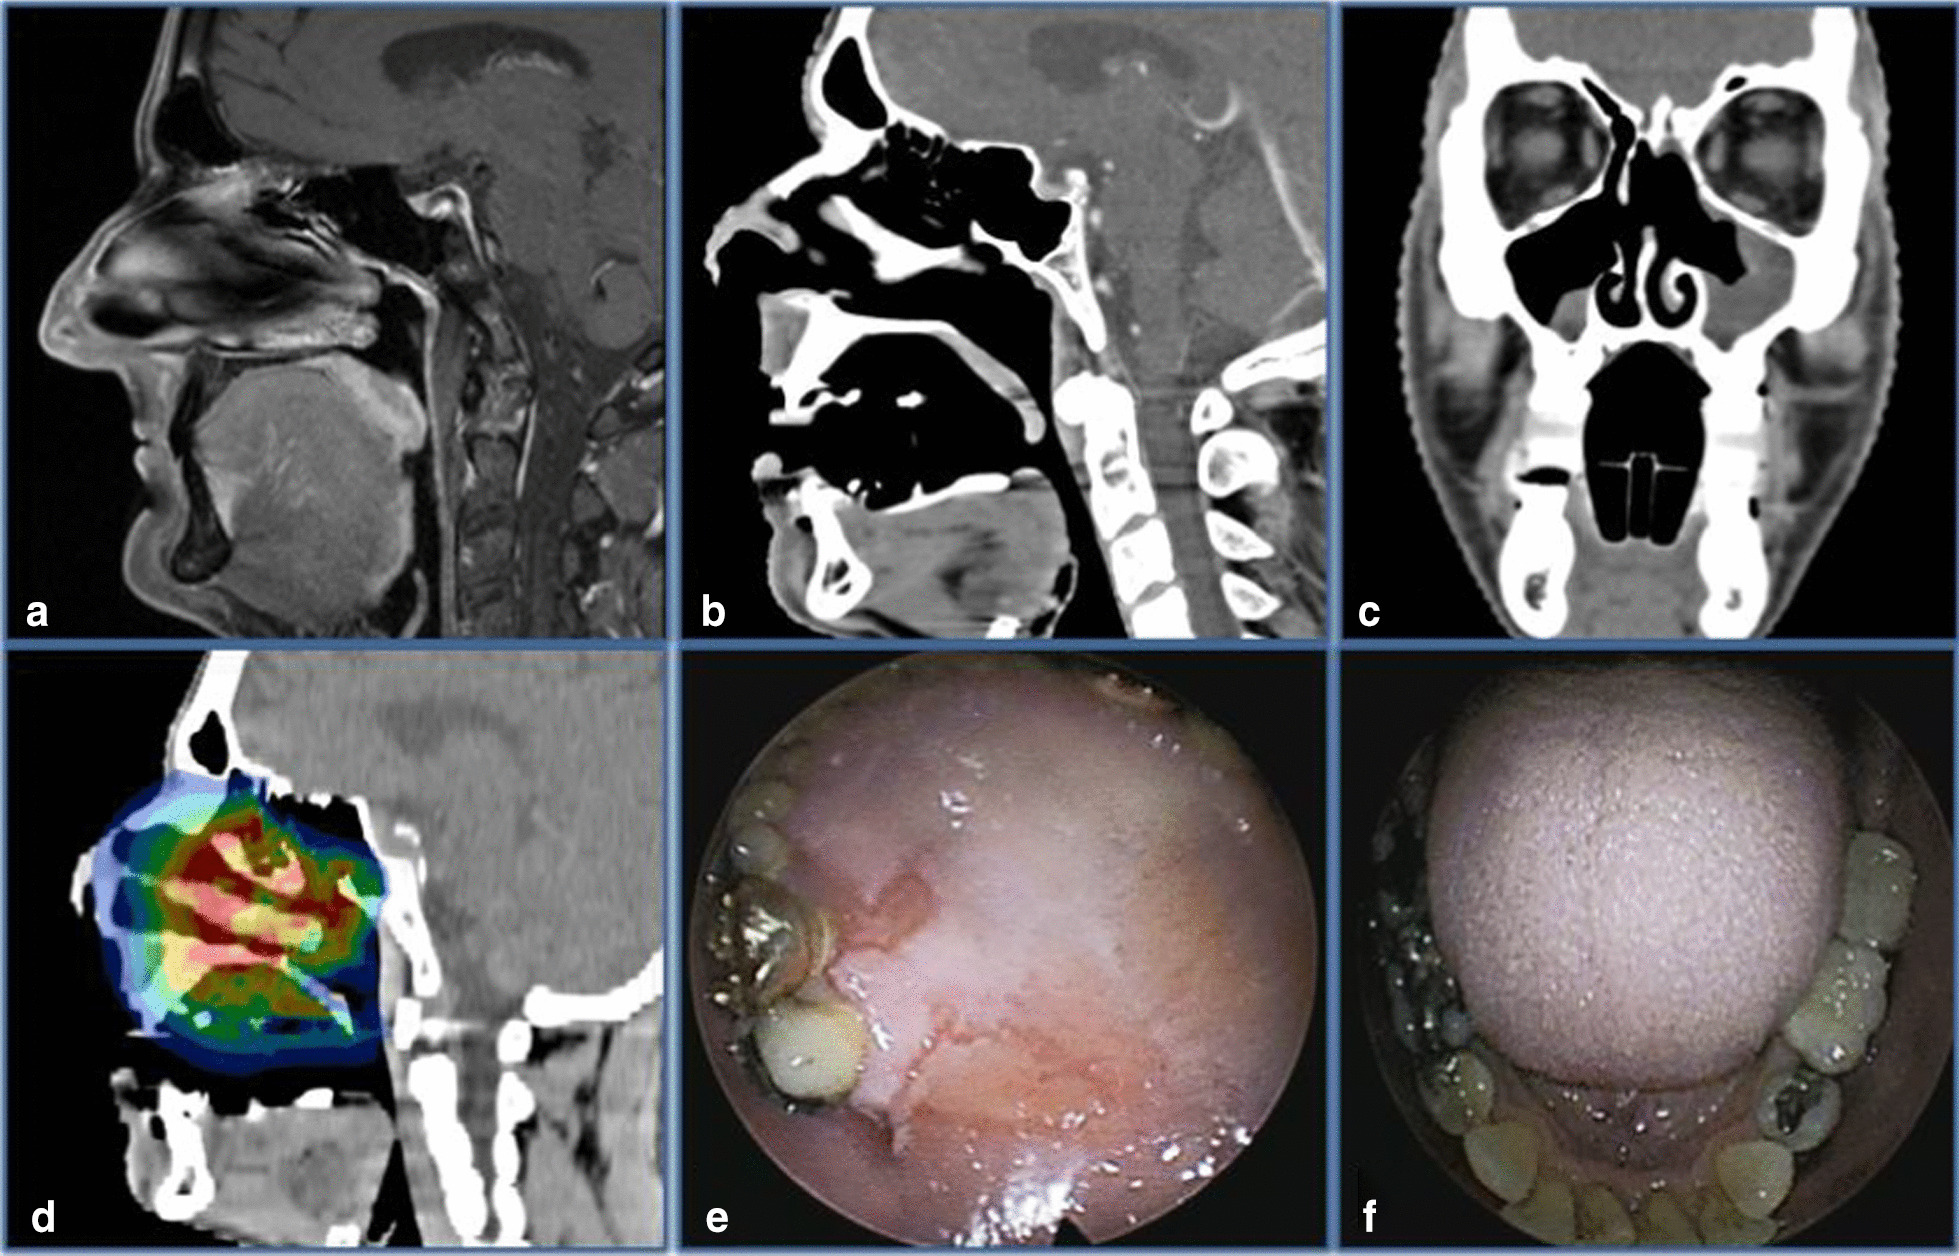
a T1 contrast-enhanced fat-saturated magnetic resonance imaging of a patient with sarcoma of the paranasal sinuses without tissue retraction device (TRD). b, c Contrast-enhanced computed tomography of the same patient with TRD. d Radiation treatment plan with TRD in situ. e Oral mucositis CTC grade III in the high-dose area of the radiation field. f Nonirritated tongue surface directly opposite the high-dose area of the radiation field
